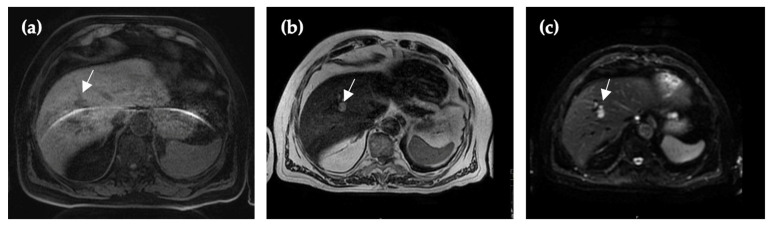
Thirty-two months after initial imaging, MRI reveals multiple hypointense lesions (arrow) in the VIII segment of the liver in T1 (a), in which no mural nodules or solid internal structures were identified. T2 (b) and DWI (c) sequences revealed hyperintense lesions with septa, which support the characteristics of lymphangioma.
